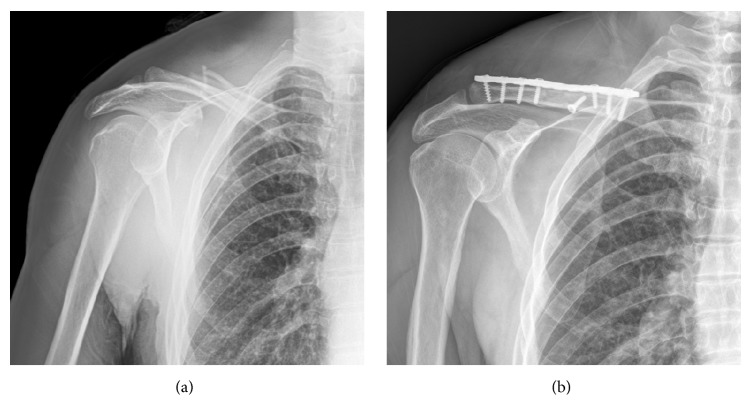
(a) Right closed comminuted midshaft clavicle fracture; (b) fracture end anatomical reduction could be seen in X-ray films after extensive incision traditional reconstruction plate internal fixation.
